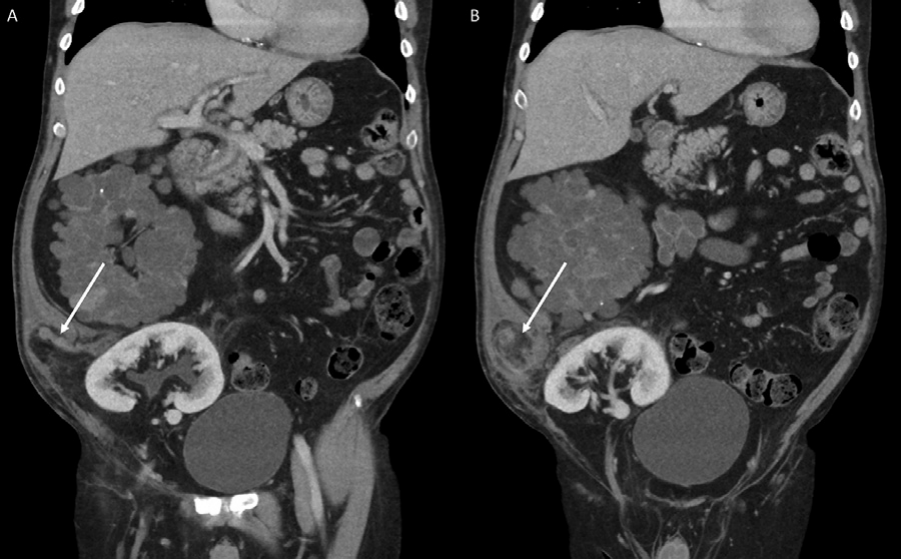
CT abdomen and pelvis with intravenous contrast administration, coronal view (Figs. A-B) demonstrates a wide neck incisional hernia in the right lower quadrant containing a tubular structure (white arrow) with associated diffuse edema in the hernial sac. The tubular structure demonstrates mild mucosal enhancement and wall thickening. Of note: The image also includes, right polycystic kidney and transplanted kidney in the right Iliac Fossa.
